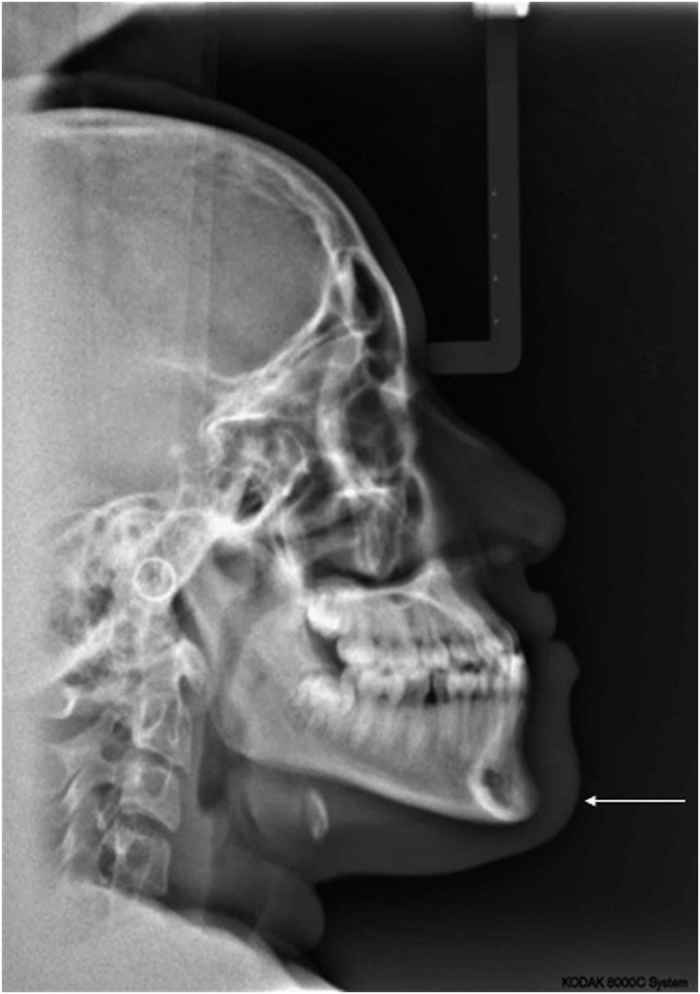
Cephalometric radiograph of affected person CPT 1 confirming a skeletal Class III malocclusion. Her mandible is prognathic (arrow).
